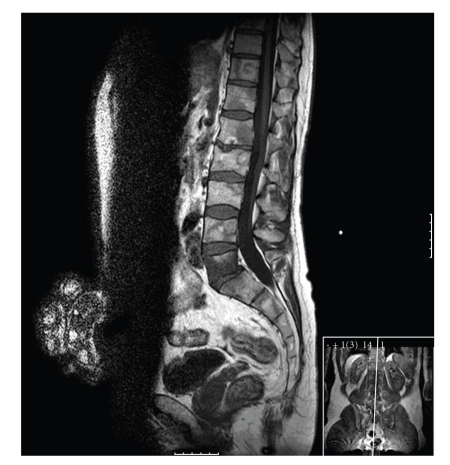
There is diffuse involvement of multiple vertebrae including L5 seen throughout the spine on T1-weighted imaging with both low and high signal.
